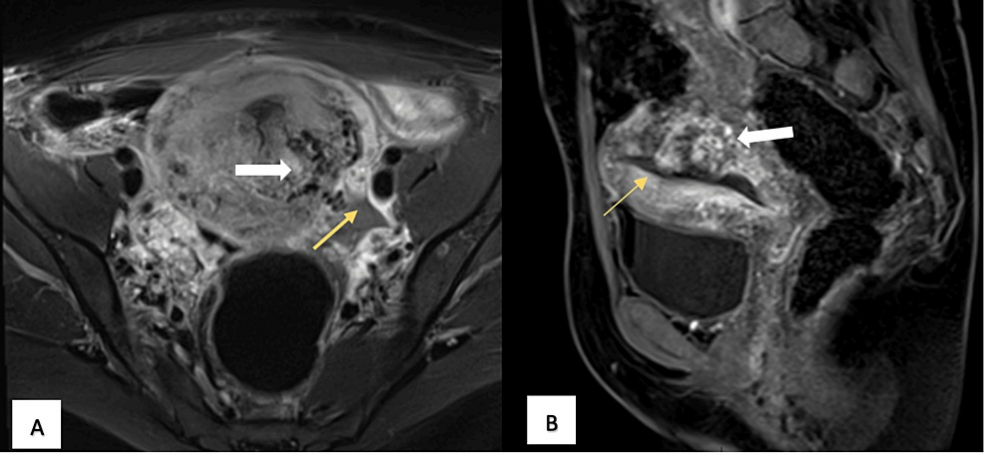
(A) Axial image, T1-weighted sequence FAT SAT with gadolinium in the early phases of dynamic acquisition, showing an enhancement of the heterogeneous appearance of the structures of the vascular nidus (white arrow); the ectasia of the pelvic vascular structures is confirmed (yellow arrow). (B) Sagittal image, T1-weighted sequence FAT SAT with gadolinium in the late phases of dynamic acquisition, showing the enhancement of aberrant vascular structures (white arrow) with the projection of vascular structures toward the endometrial cavity (yellow arrow). FAT SAT: fat saturation
